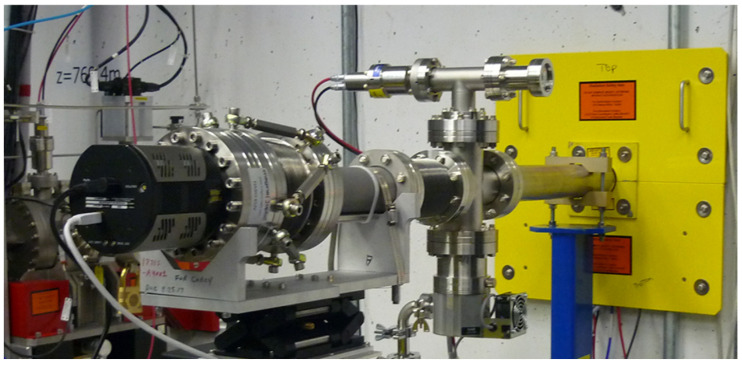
Soft X-ray wavefront sensor attached to the soft X-ray beamline at the Linac Coherent Light Source (LCLS). Reprinted with permission from [22].
